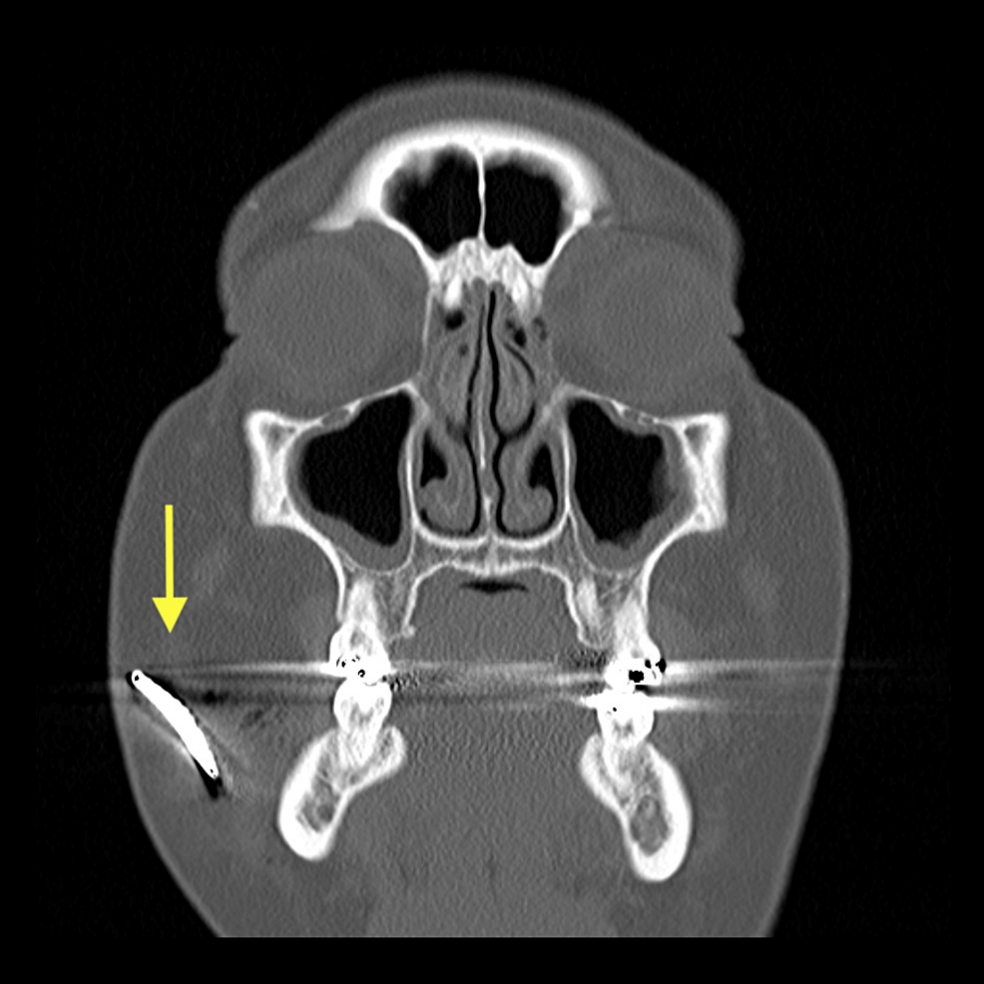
CT maxillofacial scan showing a metallic object embedded in the subcutaneous soft tissues overlying the right maxilla in the coronal view
